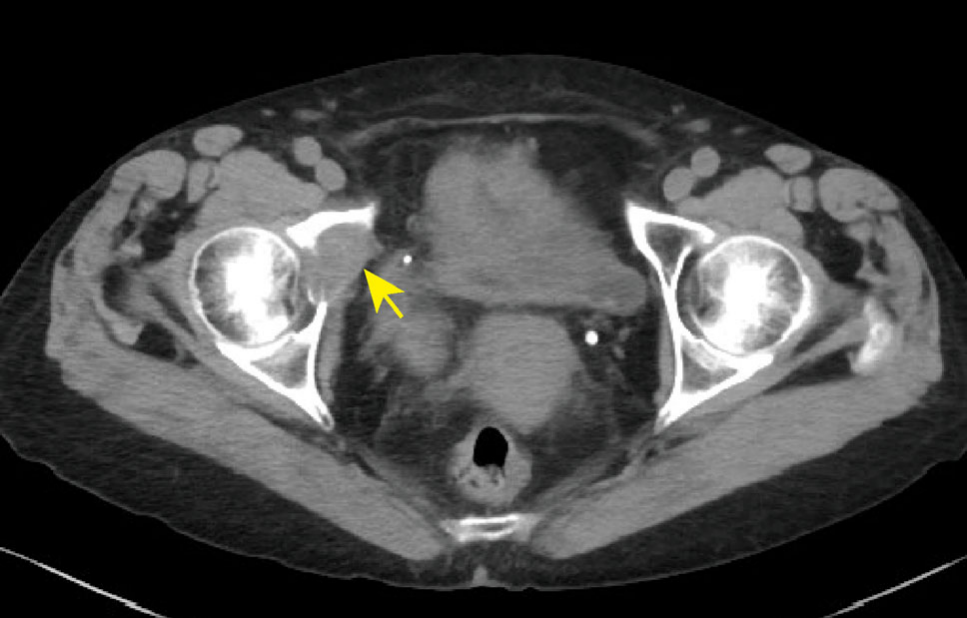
Right iliac bone metastasis. In April 2018, metastasis in the iliac bone was observed by CT scan (arrowhead).
